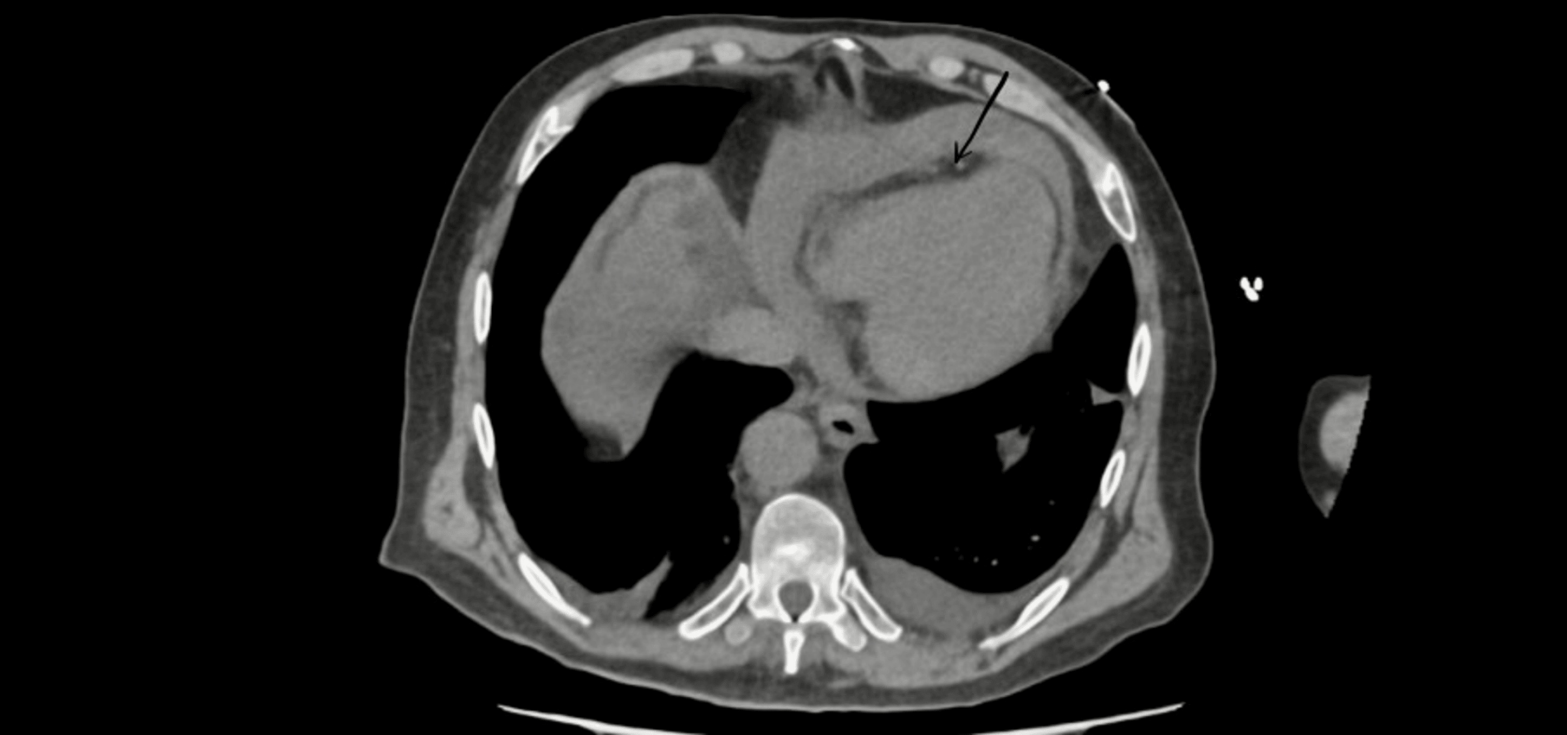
Pericardial effusion (black arrow) on CT scan. This was confirmed intraoperatively to be a hemopericardium.
